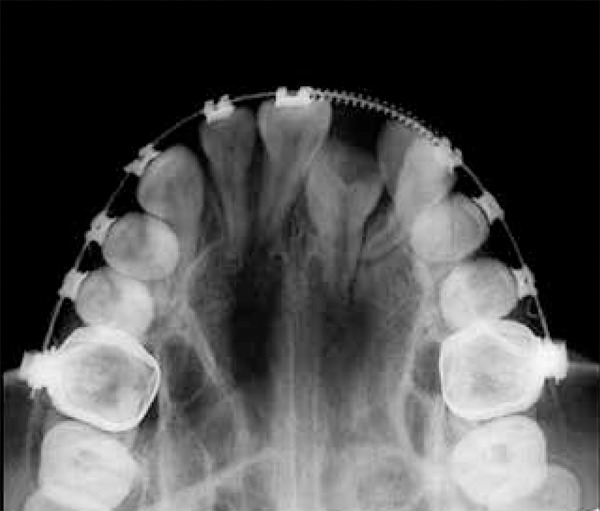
Occlusal radiograph revealing impaction of tooth #21 with complete root
